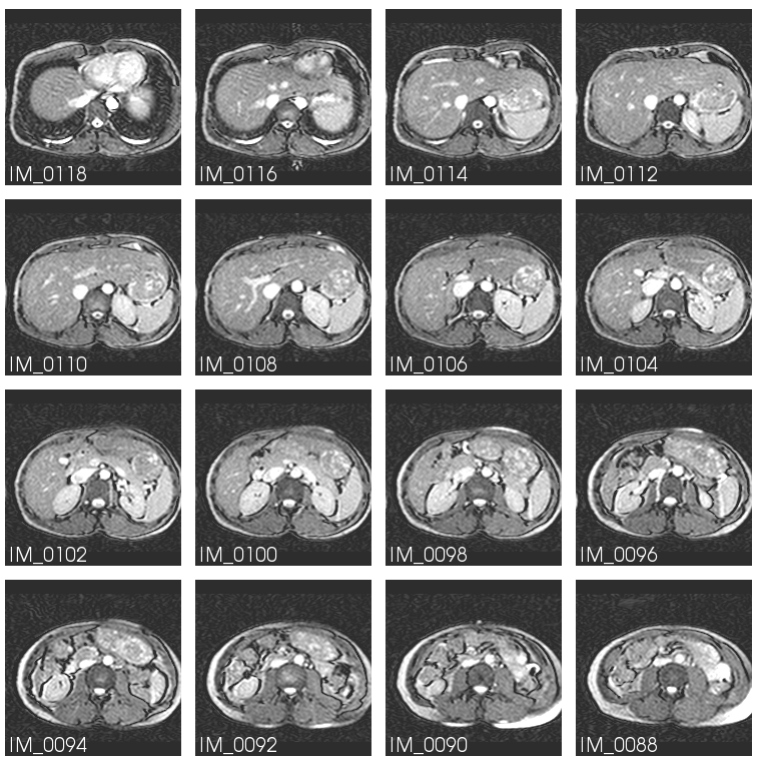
MR image stack of the torso. A subset of the survey series (301). IM_0090 and IM_0108 show the marker pills used as spatial reference.
